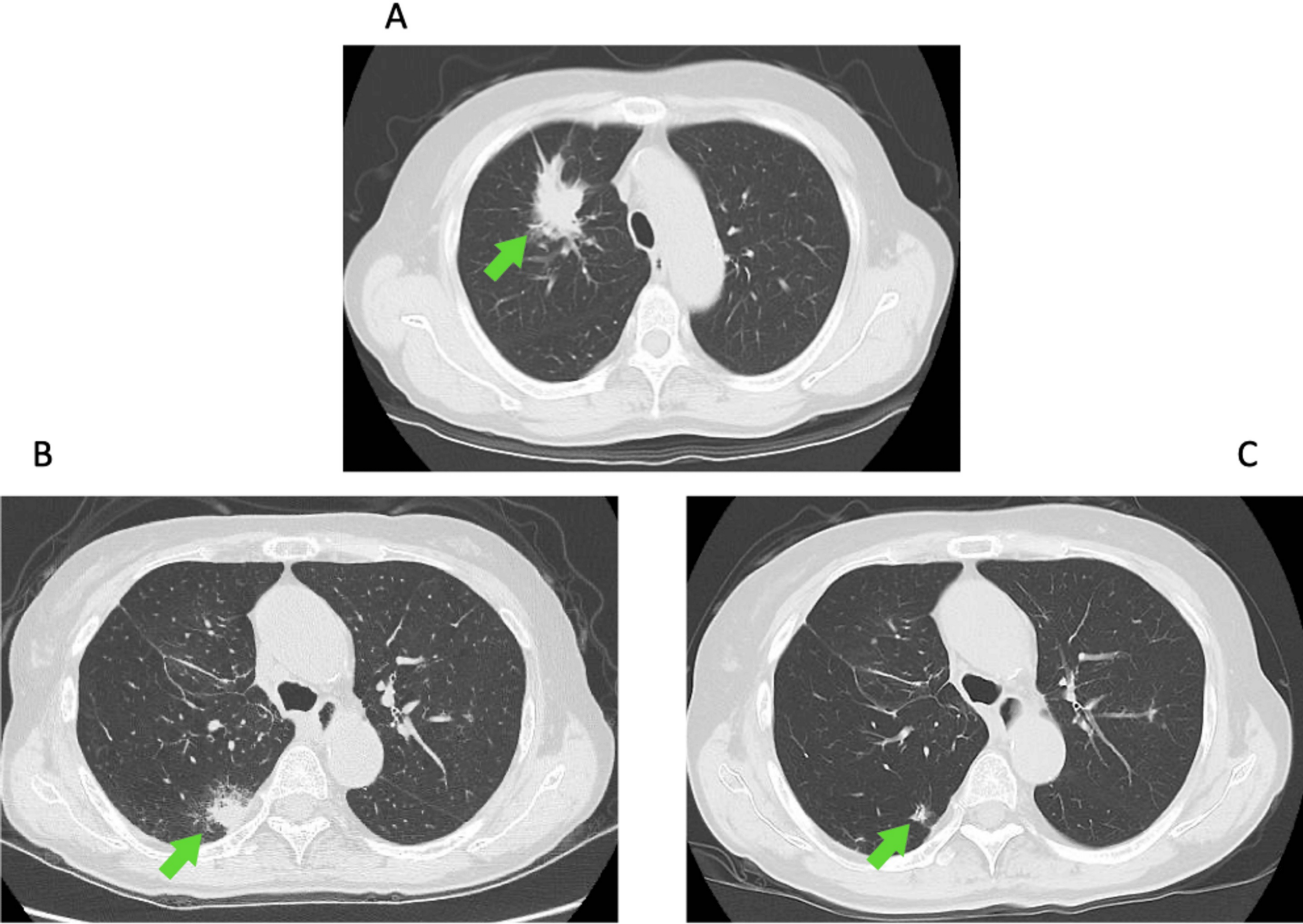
Representative CT images. A: Chest CT image pre-surgery reveals a mass shadow (arrow) in the right lower lobe.  B: Chest CT image at 14 months post-surgery reveals a recurrent nodule (arrow) in the right lower lobe. C: Chest CT image at 6 months post-chemotherapy initiation depicts a smaller nodular shadow (arrow).
